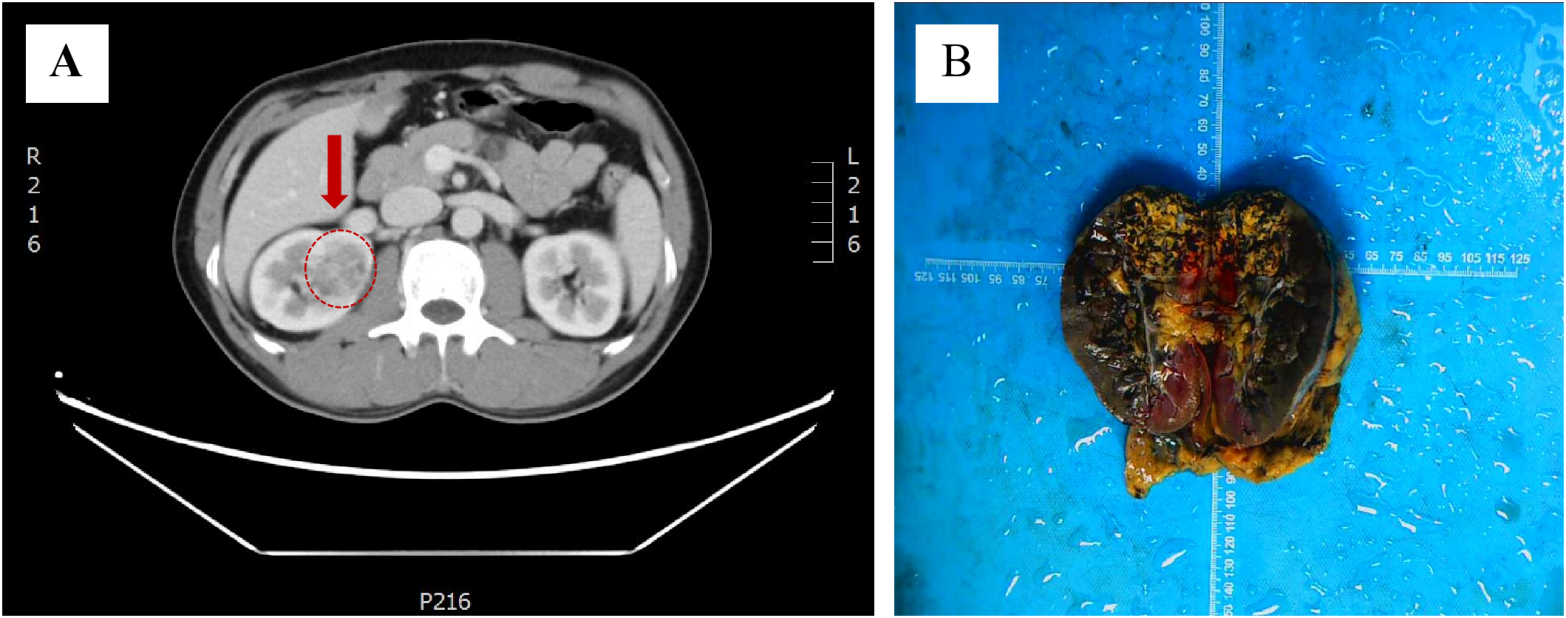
Discovery of *ELOC*(*TCEB1*)-RCC. (A) CT scans showed rounded low-density shadows in renal parenchyma. (B) Gross observation of *ELOC*(*TCEB1*)-RCC.
